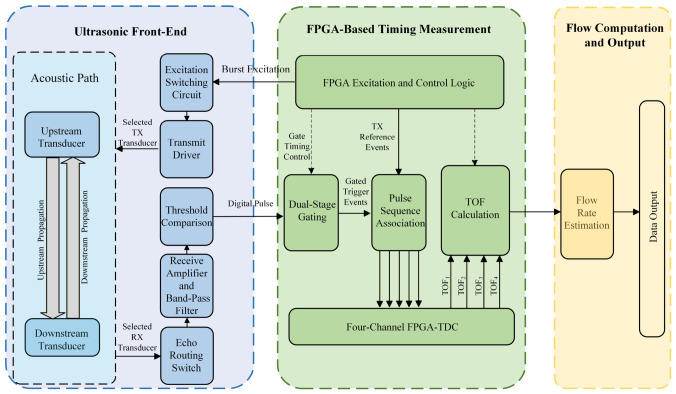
Overall architecture of the proposed FPGA-TDC-based ultrasonic TOF extraction system.
